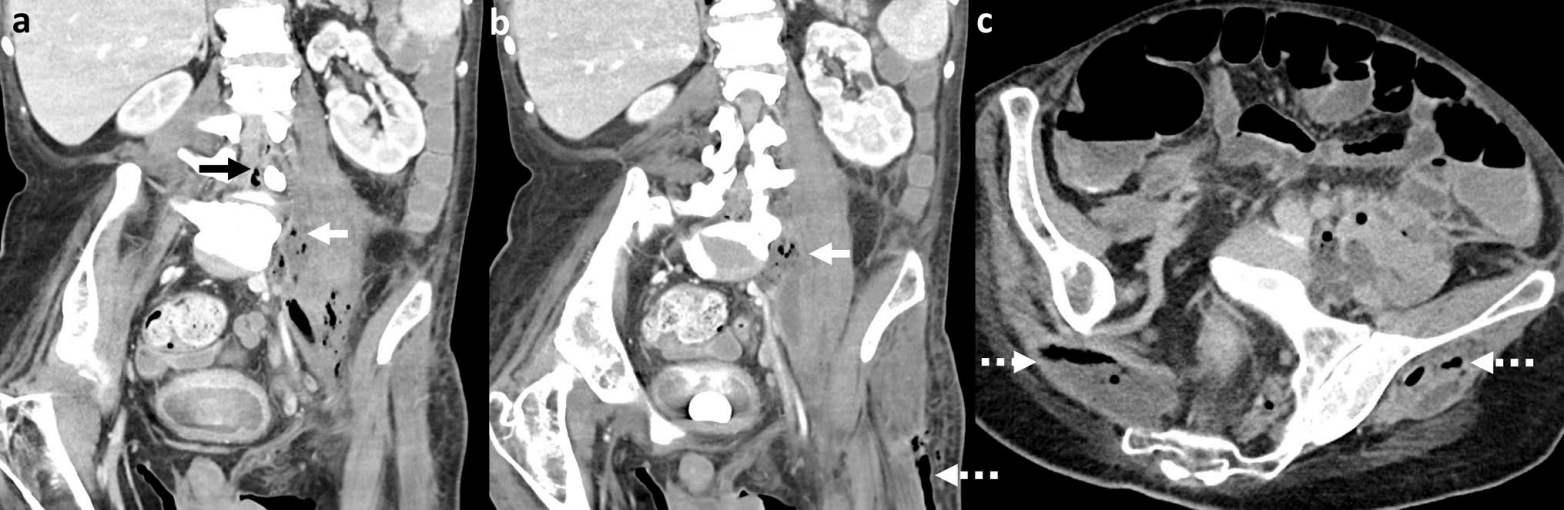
Emphysematous Iliopsoas Abscess. A 44-year-old paraplegic with pressure ulcers, urinary tract infection, and fever. CT images show left iliopsoas abscess with air foci within (solid white arrows a, b). The abscess extends to the lumbar spinal canal (black arrow, a). Air also tracks down to the left thigh (dotted white arrow, b), There are additional bilateral gluteal intramuscular abscesses (dotted white arrows, c). Percutaneous drain was placed into the iliopsoas abscess, and fluid cultures showed an anerobic polymicrobial infection
